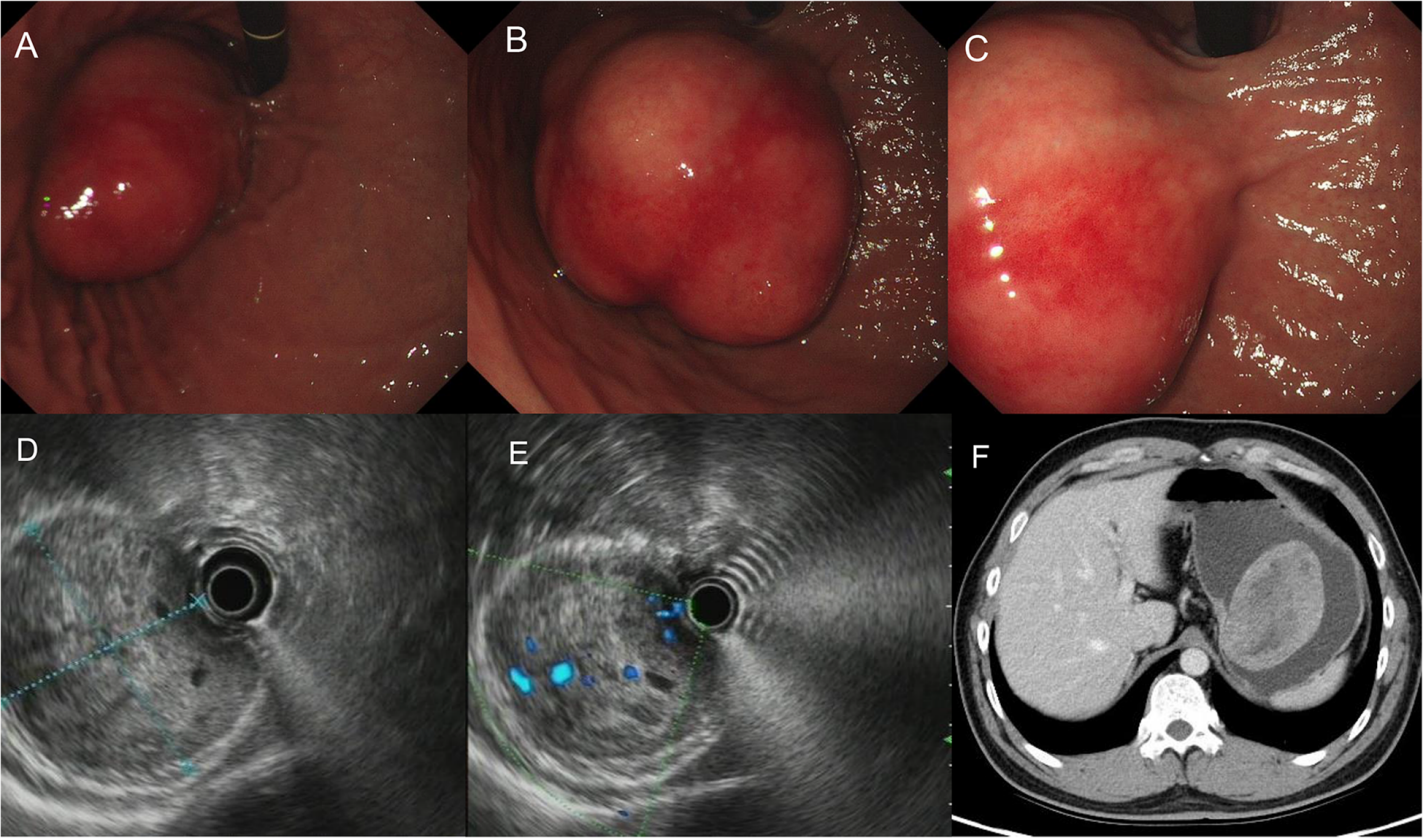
a, b and c Upper gastrointestinal endoscopy revealing a submucosal tumor at the lesser curvature of the esophagogastric junction. d and e Endoscopic ultrasonography revealed a round, low-echoic mass. f The enhanced computed tomography (CT) scan shows a mass abnormal enhancement, and the lesion is seen on the side of lesser curvature of gastric body (9.5 cm × 6.3 cm) at the esophagogastric junction
